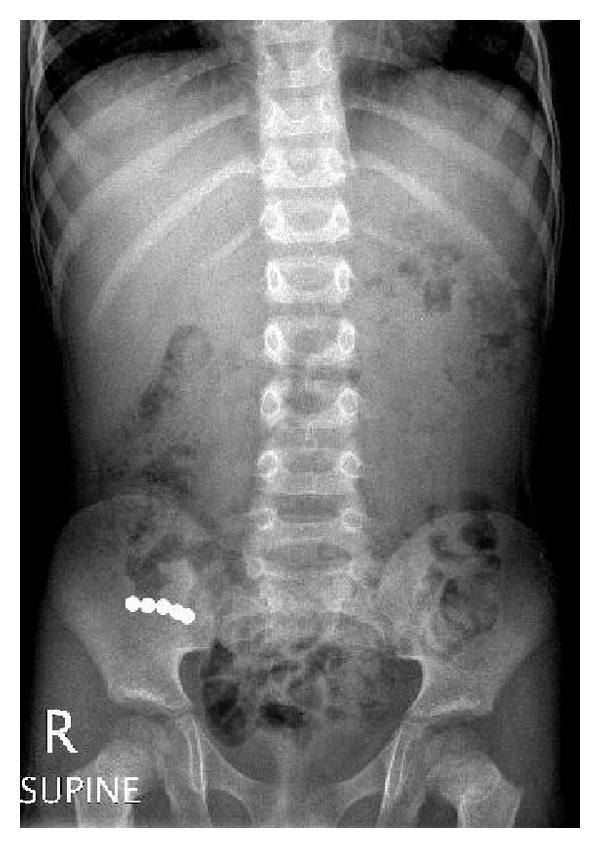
Plain X-ray of abdomen of 4-year-old boy showing ingested 5 pieces of rare-earth magnets joined together due to strong magnetic force.
